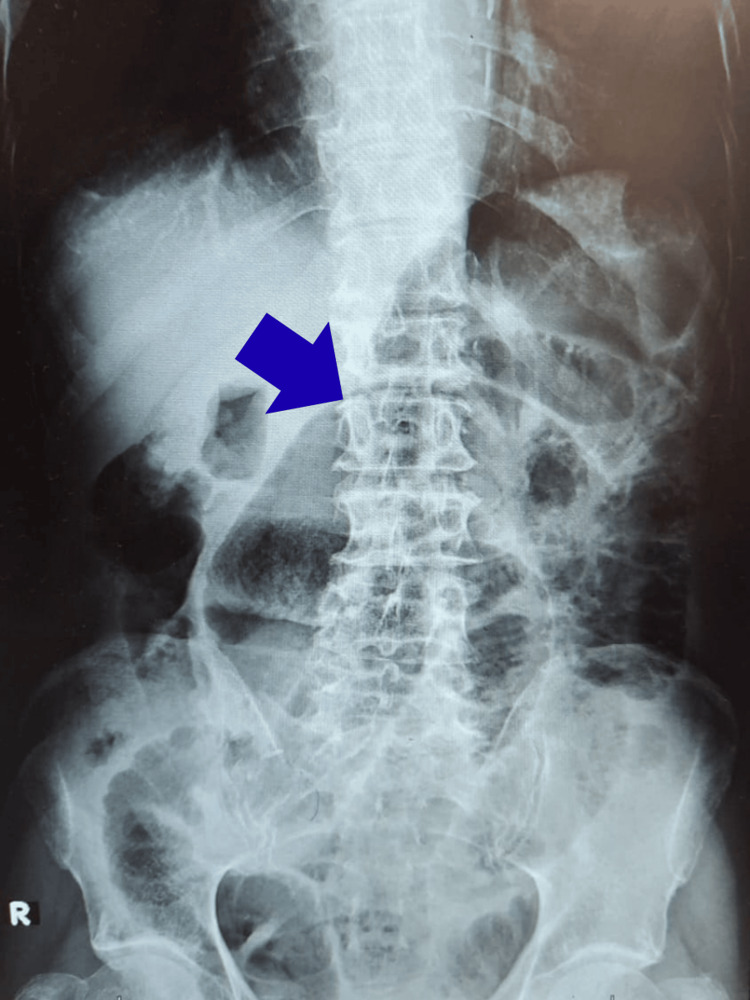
Supine abdominal X-ray showing dilated bowel loops. The blue arrow indicates a dilated bowel loop.
